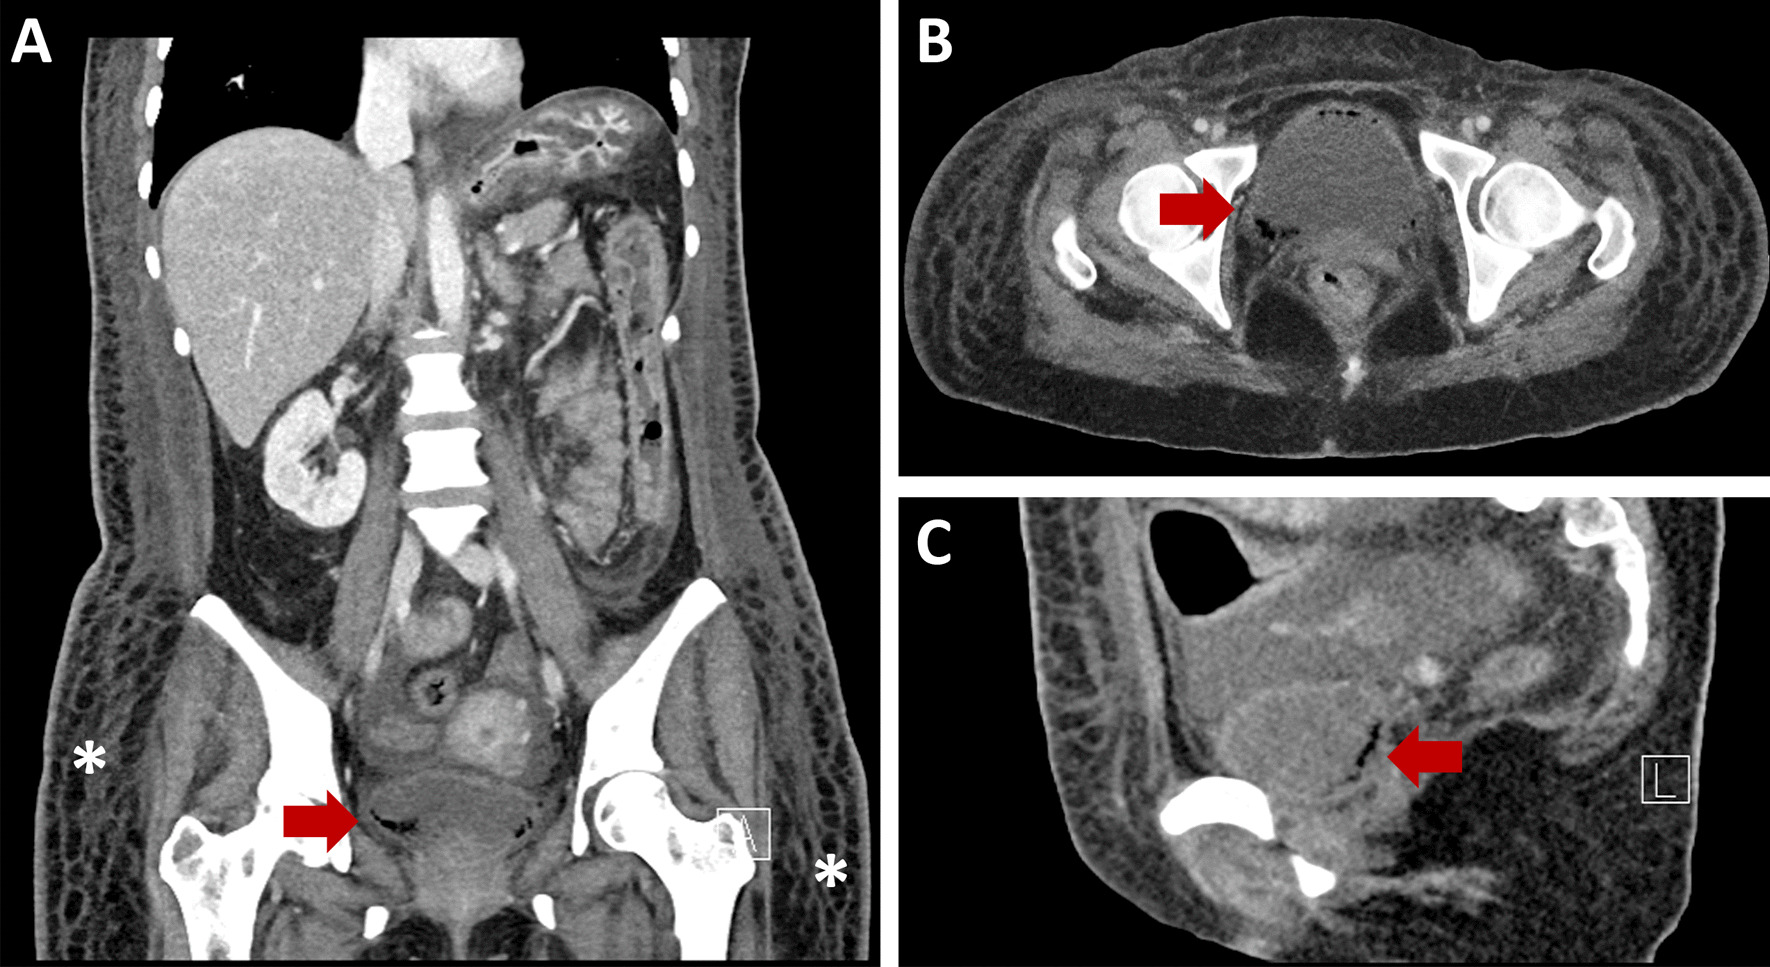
Non-contrast enhanced CT scan of the abdomen and pelvis showing diffuse anasarca (*) and emphysematous cystitis (red arrow) in the a coronal plane. Emphysematous cystitis is also shown in the b axial and c sagittal planes
